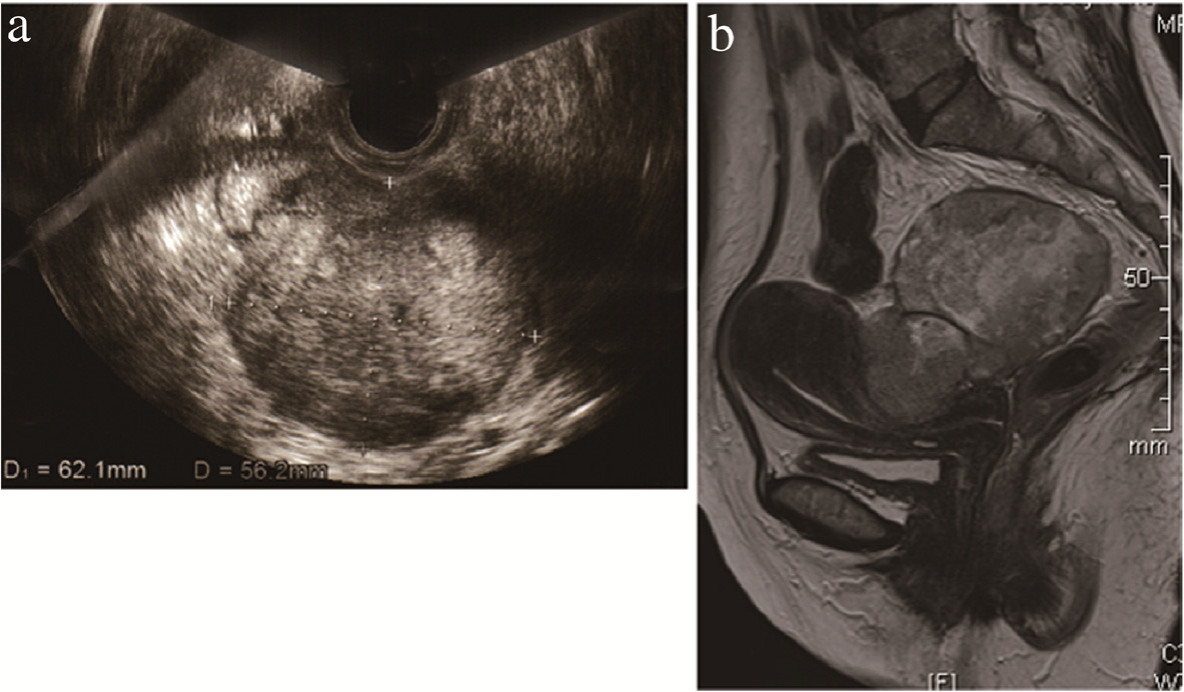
Clinical imaging of the tumor. a. Vaginal ultrasonography revealed a 6 cm heterogeneous tumor located in the pouch of Douglas, with a suspected left ovarian involvement. b. T2-weighted MRI revealed an 8 cm heterogeneous tumor located at the posterior (intestinal) surface of the uterus, with a suspected rectal invasion.
